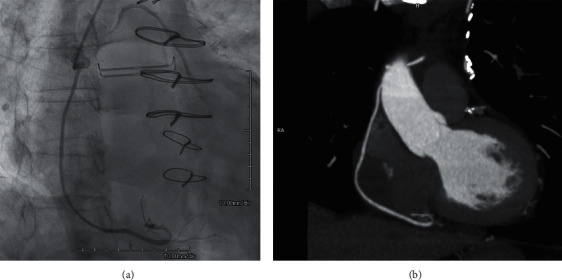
Spontaneous closure of pseudoaneurysm. (a) During left heart catheterization approximately four weeks after initial presentation, there was no detectable pseudoaneurysm, consistent with spontaneous closure. (b) On follow-up CT imaging approximately three and a half months after initial presentation, there was again no detectable pseudoaneurysm and the mediastinal hematoma had decreased in size.
